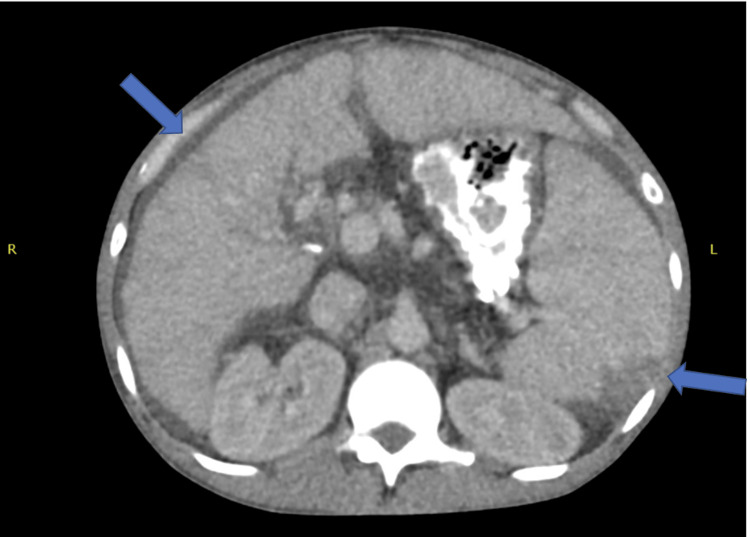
Computed tomography revealing a wedge-shaped hypodensity in the spleen representing infarction, moderately large amount of ascites surrounding the liver, and irregularity of the liver contour demonstrating cirrhosis of the liver.
